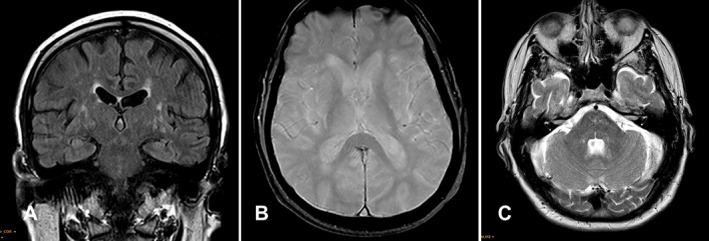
The coronal T2 FLAIR sequence (A) shows widening of perivascular spaces and white matter hyperintensities in the white matter of centrum semiovale and basal ganglia suggestive of cerebral SVD. Axial gradient echo image at the level of the basal ganglia (B) demonstrates a normal signal without signal dropout, and the axial T2‐weighted image (C) at the level of the pons, middle cerebellar peduncles, and cerebellar hemispheres shows patchy non‐specific foci of high T2 signal in the pons.
